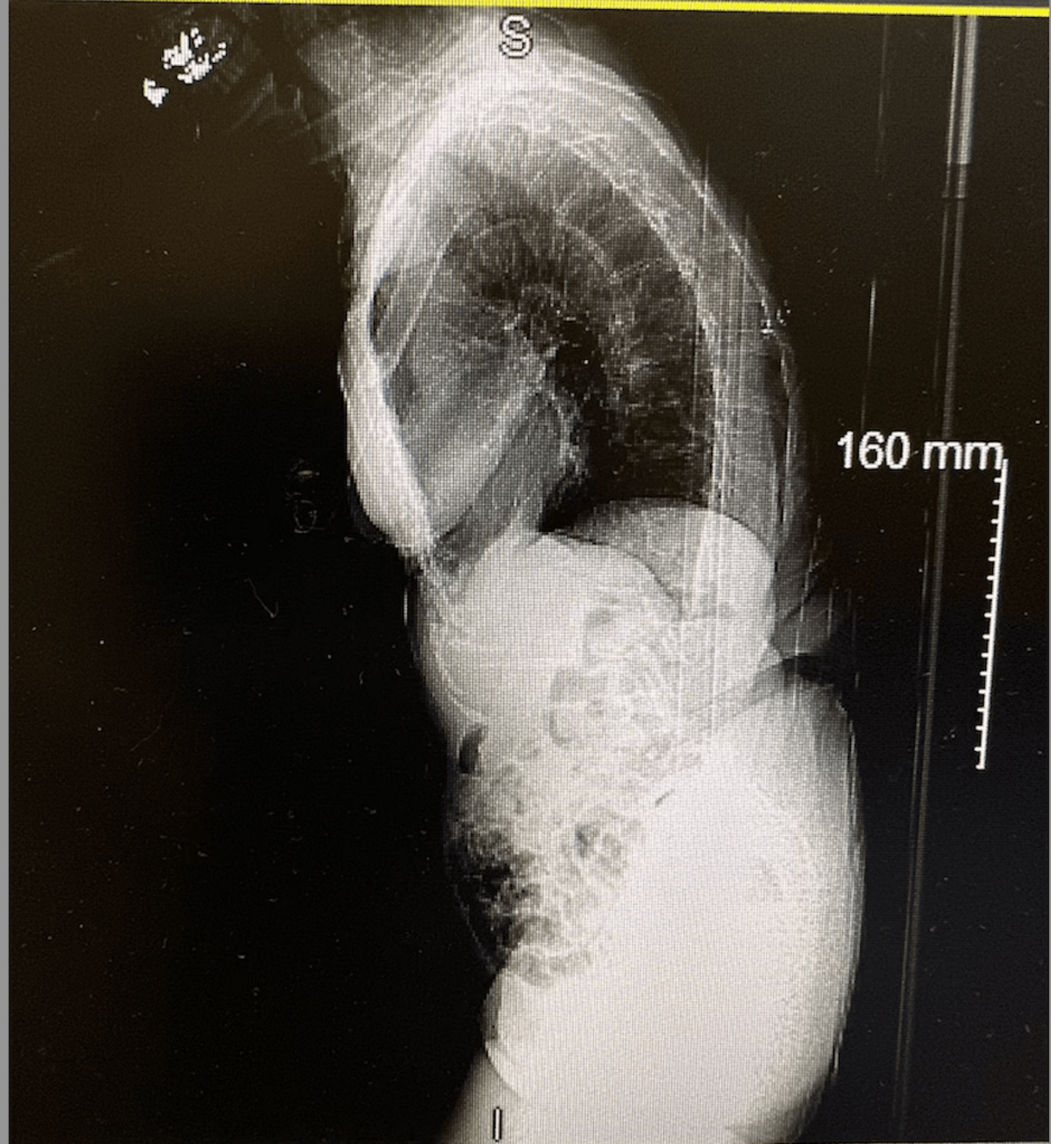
Lateral view CT scan of the thoracolumbar spine CT: computed tomography
